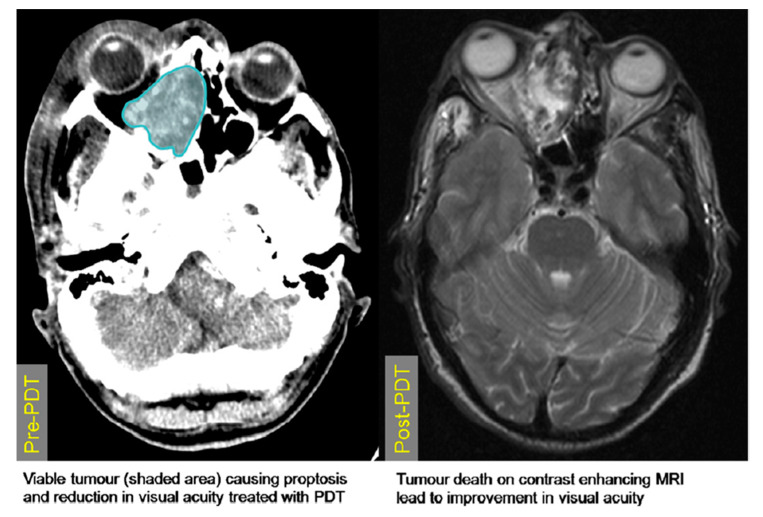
CT scans pre- and post-PDT of a mass located in the sinonasal area and extending into the retrobulbar space, affecting vision and causing proptosis. The post-PDT CT displays a reduction in bulk of the mass, leading to an improvement in visual symptoms. Reprinted with permission from ref. [43]. Copyright 2010 Photodiagnosis Photodyn. Ther.
